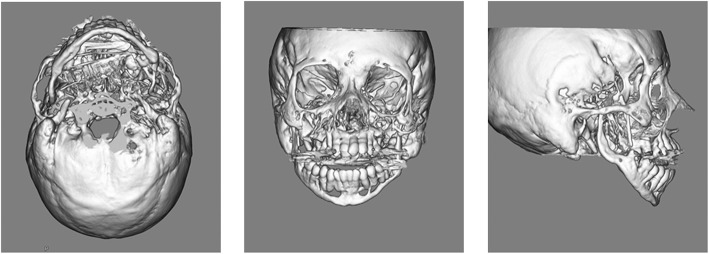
Computed tomography imaging. Imaging shows axial and coronal thinness of the alveolar bone, anterior wall of the maxillary sinus in the maxilla, and the entire mandibular (condyle, angle, body, and alveolar) bone
